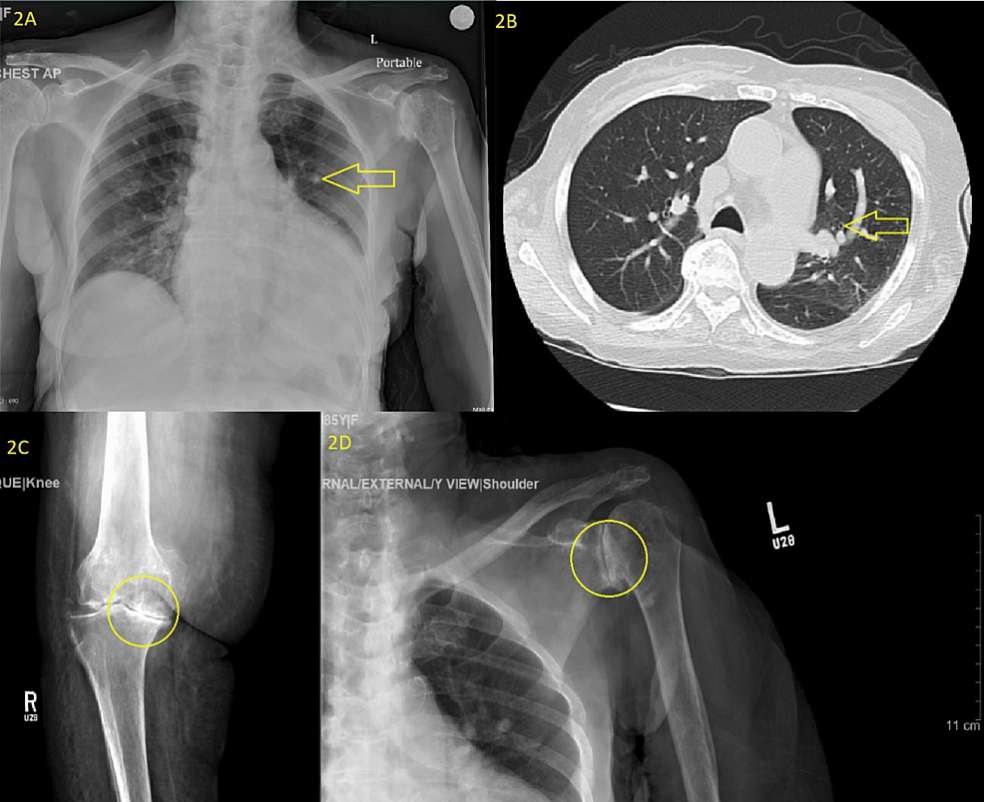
Diagnostic imaging on admission. A: Chest X-ray of acute pathology with a calcified granuloma (arrow). B: CT of the chest: centrilobular emphysema (arrow). Four-chamber cardiac enlargement and no lung mass, suspicious nodule, or active infiltrate or congestion. C: X-ray of the right knee showed severe osteoarthritis (circle). D: No acute pathology. Severe glenohumeral osteoarthritis (circle).
